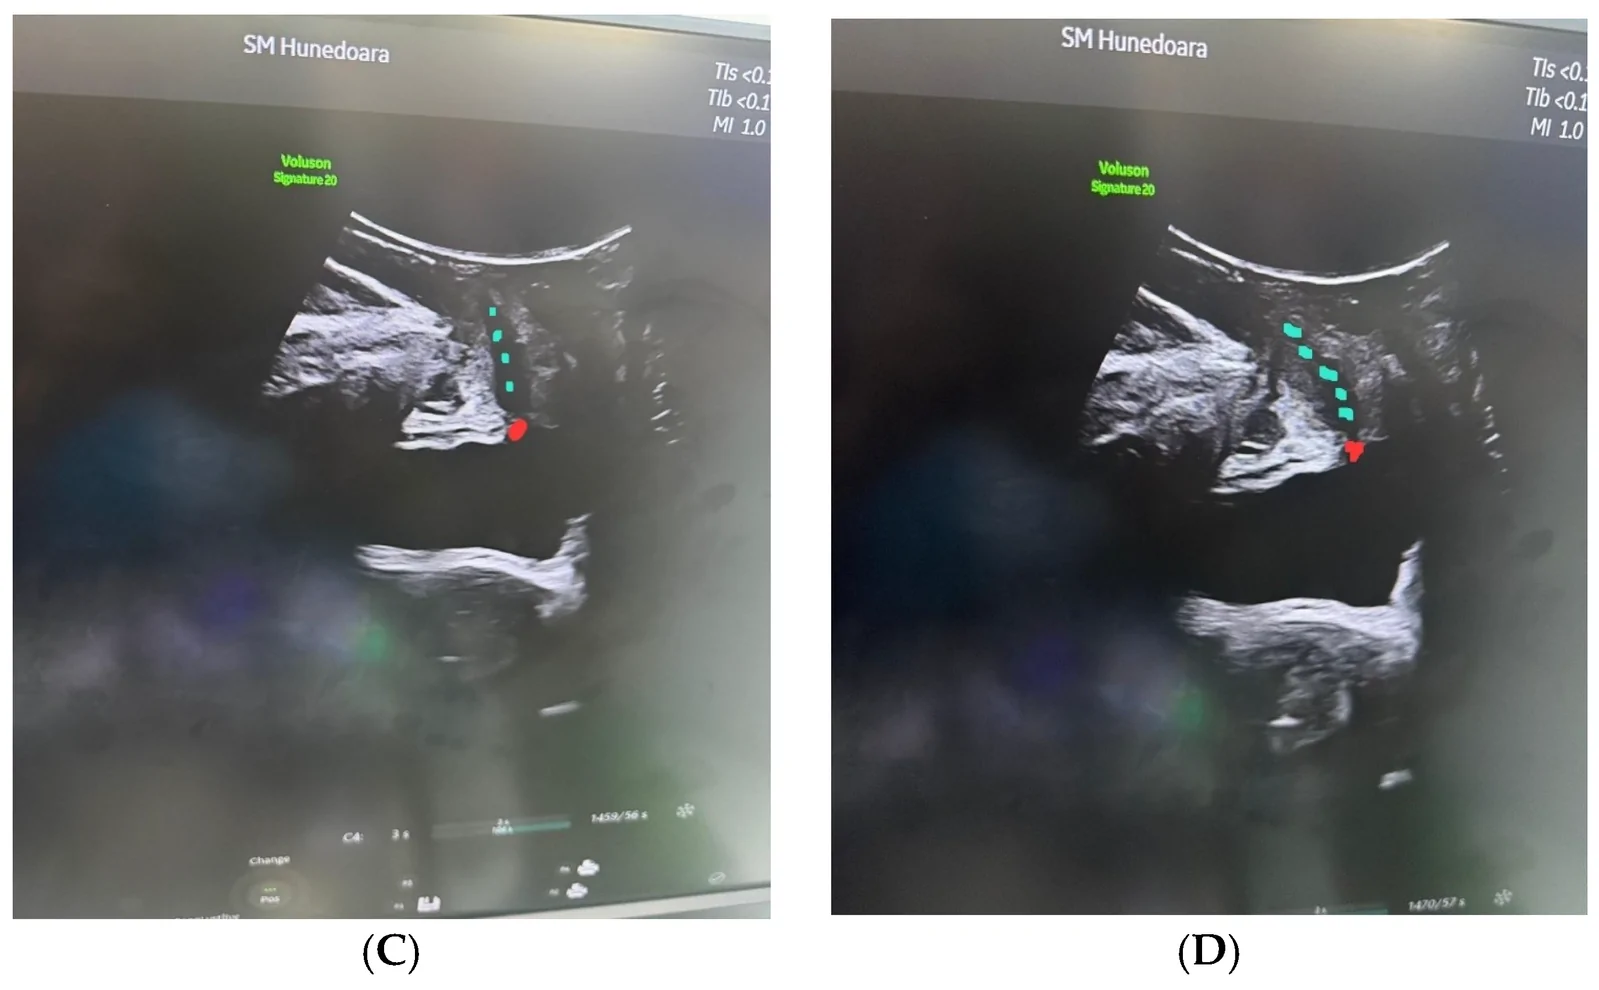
TPUS images of SUI cases: (A,B) the funel shape of urethra during the Valsalva maneuver in midsagital plane; (C) the urethra (green) and bladder neck (red) at rest; (D) urethral mobility and bladder neck descent during Valsalva manoever in a SUI case. (Red and blue is the Doppler signal in the green frame).
